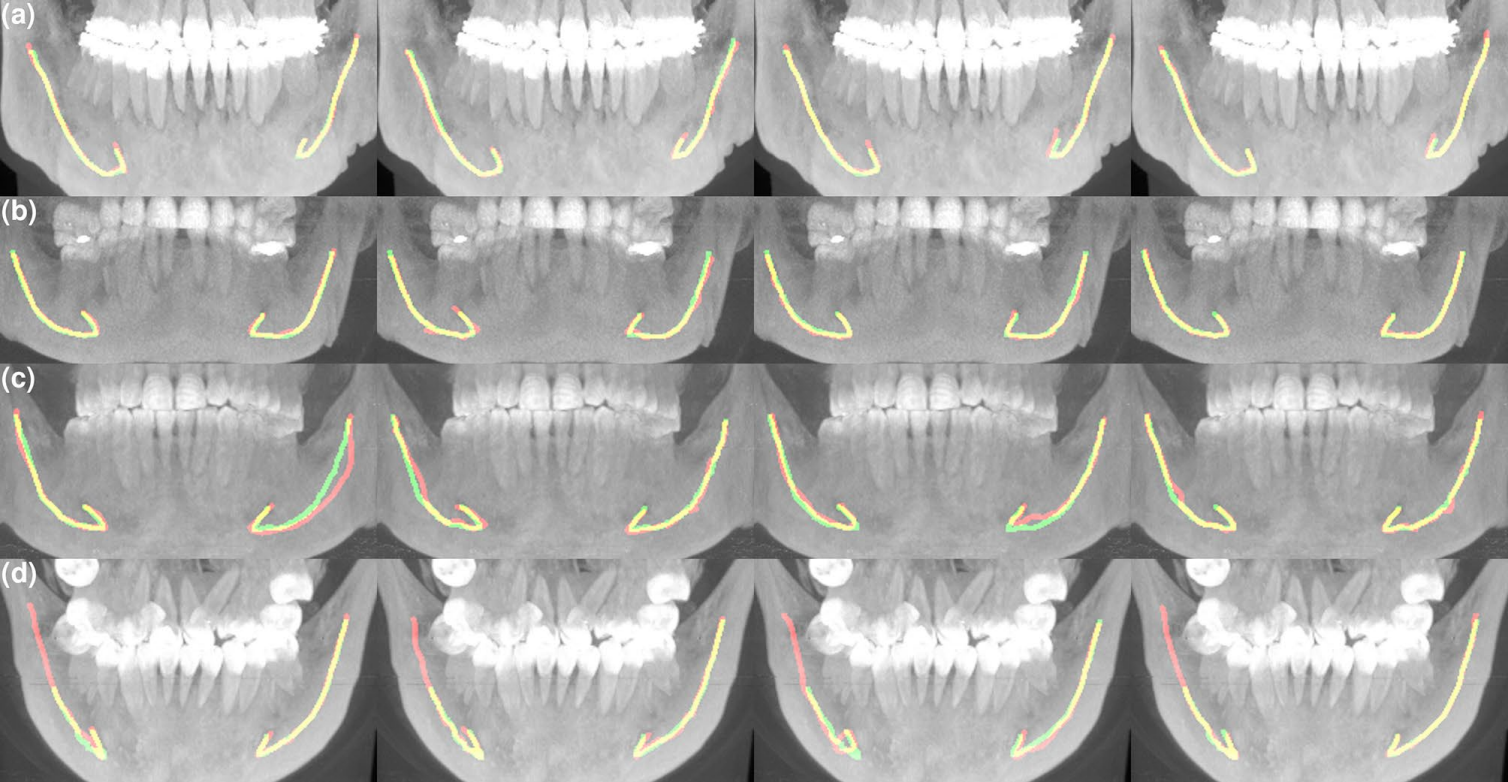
Maximum intensity projection of CBCT with overlaid expert and DLS segmentations. Every image on each column is annotated by the same expert, shown in red, DLS annotation shown in green, and overlap shown in yellow. (a) GiANO HR with 0.3 mm spacing, (b) a DentiScan with 0.4 mm spacing, and (c,d) DentiScan with 0.25 mm spacing, (a,b) Low interobserver variability (IV) and low DLS to expert variability (DV) on a scan with no heterogeneities and the both canals were labelled as Clear. (c) High IV with a scan labelled with difficult bone structure (DBS) and the left canal was labelled Unclear. (d) High DV where the DLS outcome was incomplete with a scan labelled with DBS, movement artefact, and the right canal labelled Unclear.
